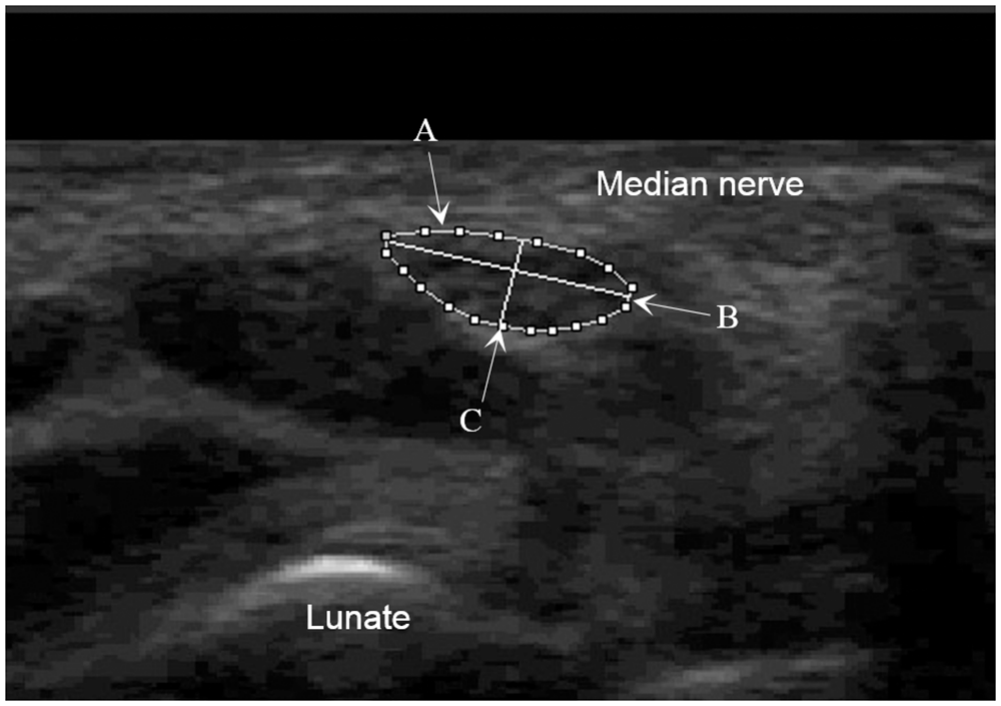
The transverse scan view of an ultrasound image of the median nerve obtained at the level of the wrist crease. The trace of the hypoechoic boundary of median nerve (A) denotes cross-sectional area of median nerve. The flattening ratio of median nerve is calculated by the ratio of B/C, where (B) denotes the major axis length and (C) denotes the minor axis length of median nerve.
